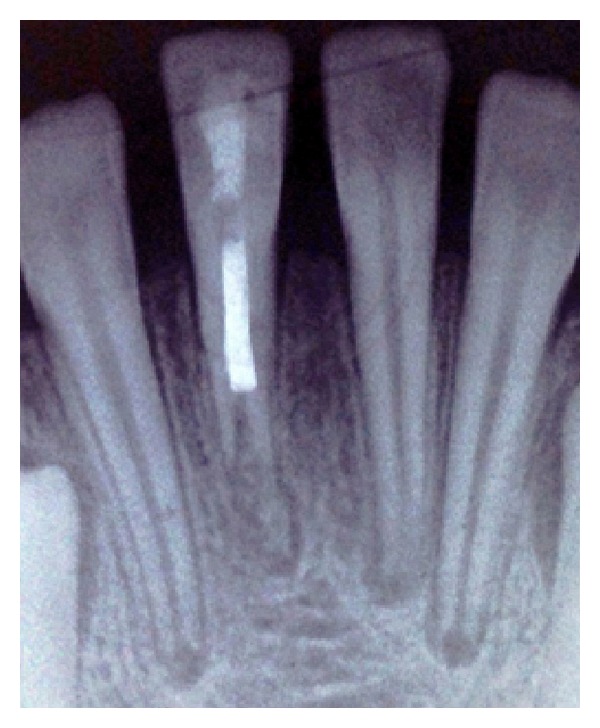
Twenty-month follow-up radiograph; continued root formation is very noticeable.
